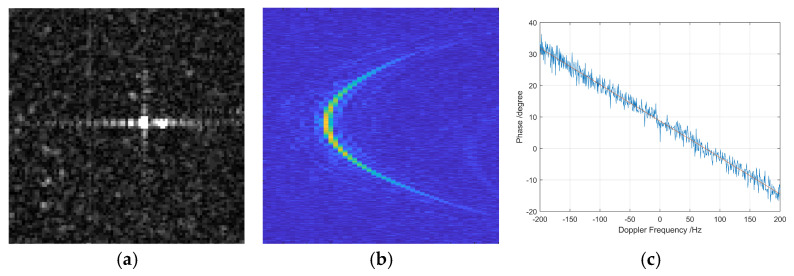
Multi-channel echo range migration curve of isolated strong point target. (a) Image slice amplitude. (b) Range migration curve after range compression in time-domain. (c) Channel phase error varying with Doppler frequency.
